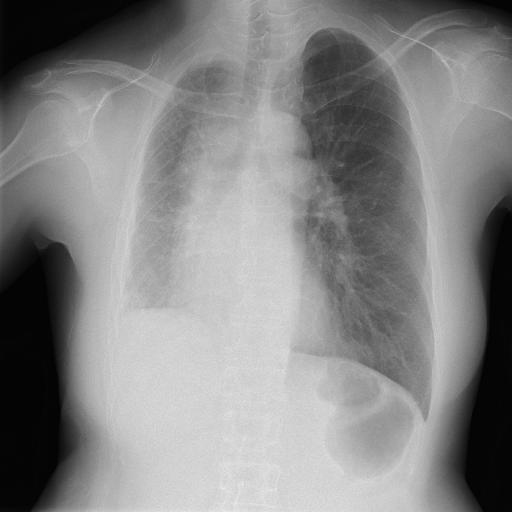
Chest radiography. Chest radiography shows decreased size of the right hemithorax, elevation of the right diaphragm, and absence of the right pulmonary artery shadow.
